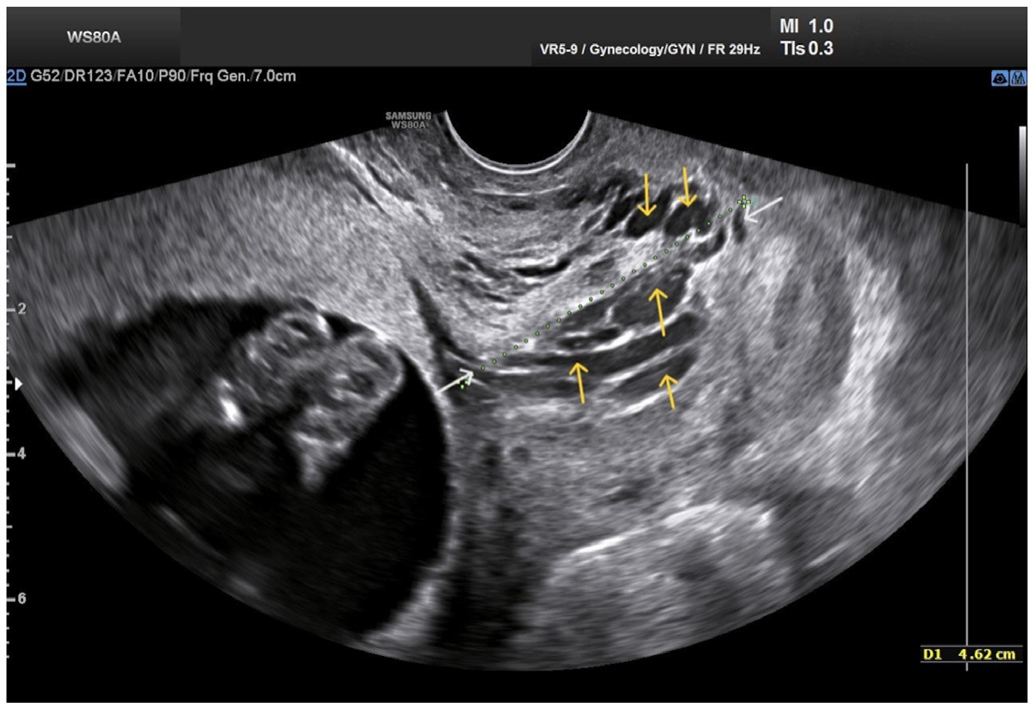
Transvaginal ultrasound imaging of cervical varices in the second trimester of pregnancy: The visualization of dilated longitudinal structures (yellow arrows) protruding through the enlarged endocervix is evident. The white arrows indicate the internal cervical os (left) and the external cervical os (right).
